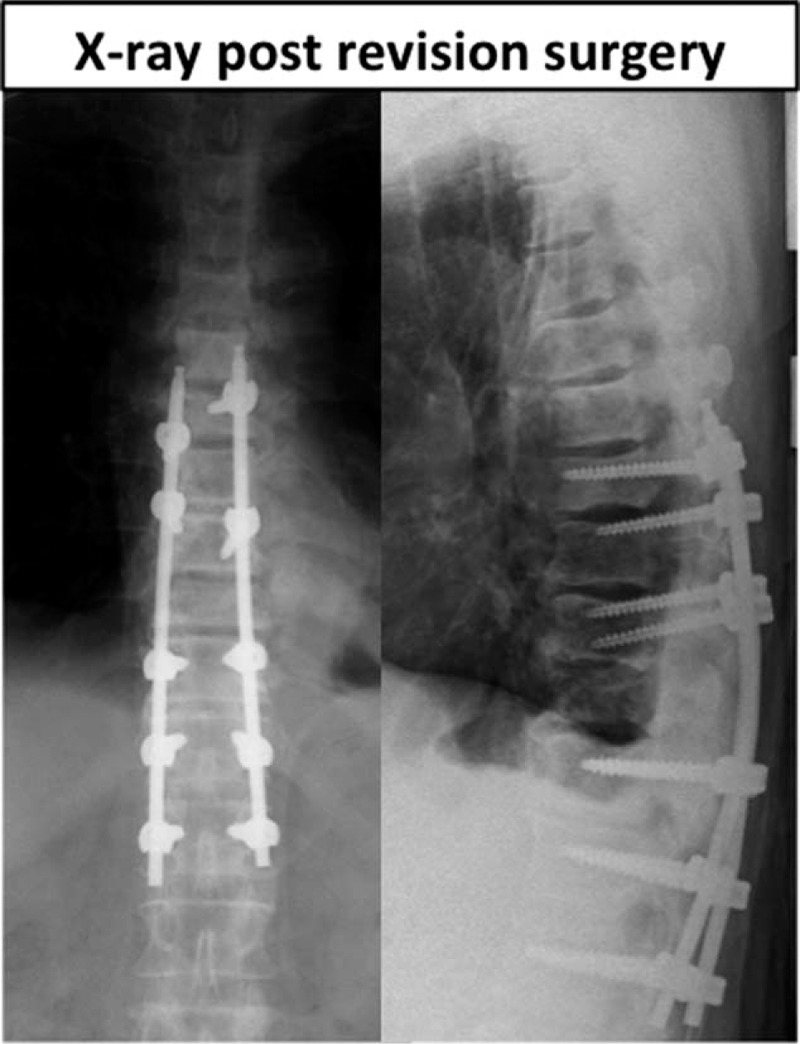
Radiographs obtained after posterior fixation surgery from T7 to L1.
